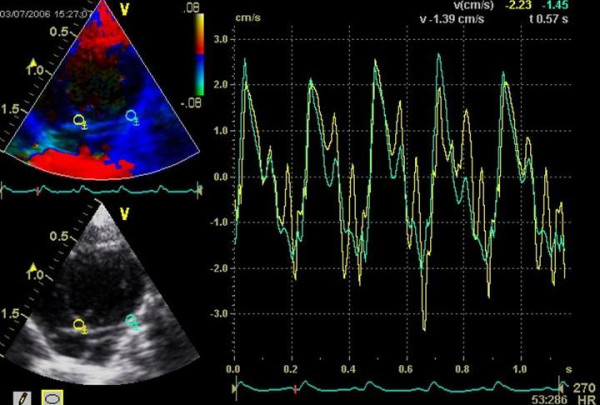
Tissue Doppler measurement from the apical view with calculation of systolic and diastolic velocities of the basal segments (septum and lateral wall).
